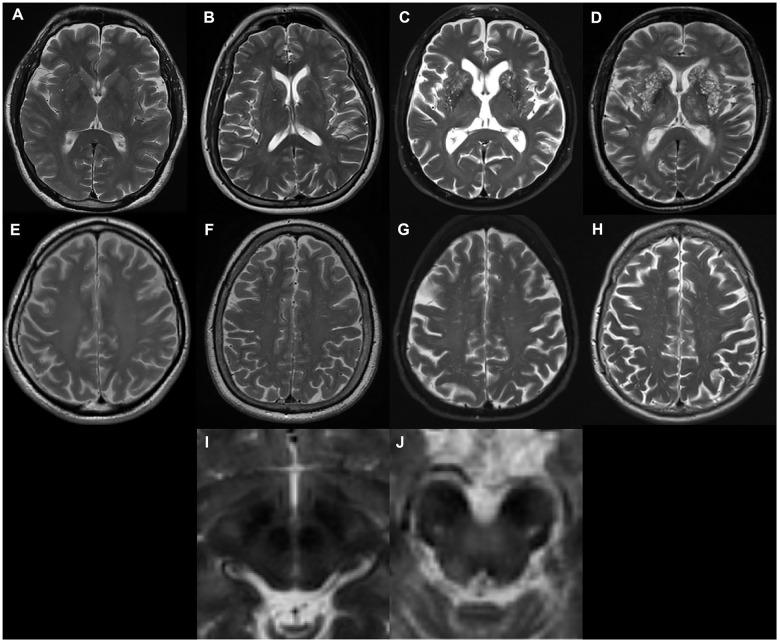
Examples of MRI-visible perivascular spaces. (A–D) Examples of MRI-visible BG-EPVS. (A) grade 1; (B) grade 2; (C) grade 3; (D) grade 4. (E–H) Examples of MRI-visible CSO-EPVS. (E) grade 1; (F) grade 2; (G) grade 3; (H) grade 4. (I) Example of no MRI-visible midbrain-EPVS. (J) Examples of MRI-visible midbrain-EPVS.
